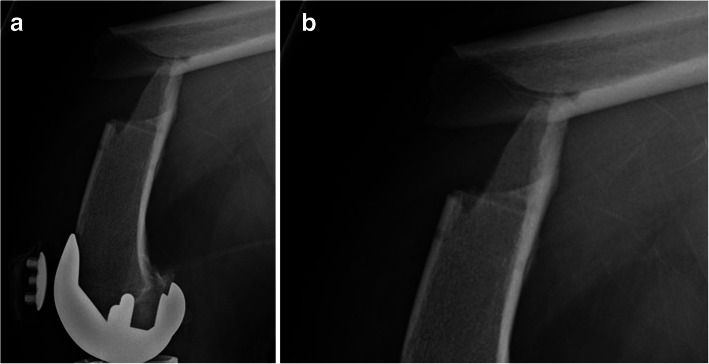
A. A lateral X-ray shows a short oblique periprosthetic femoral fracture. B. A close-up view shows the fracture originated from the stress riser left by the diaphyseal pin hole
